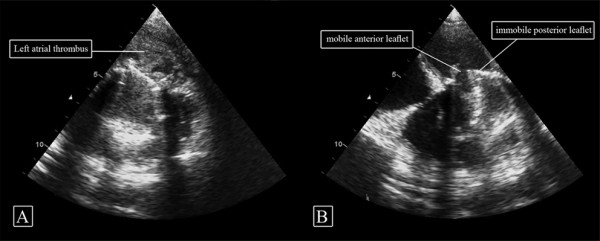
Pre-treatment transthoracic echocardiography (TTE). (A) Left atrial thrombus adherent to mitral valve prosthesis and (B) TTE showing (with arrows) the two leaflets of prosthetic mitral valve (anterior leaflet mobile while posterior leaflet thrombosed and immobile).
